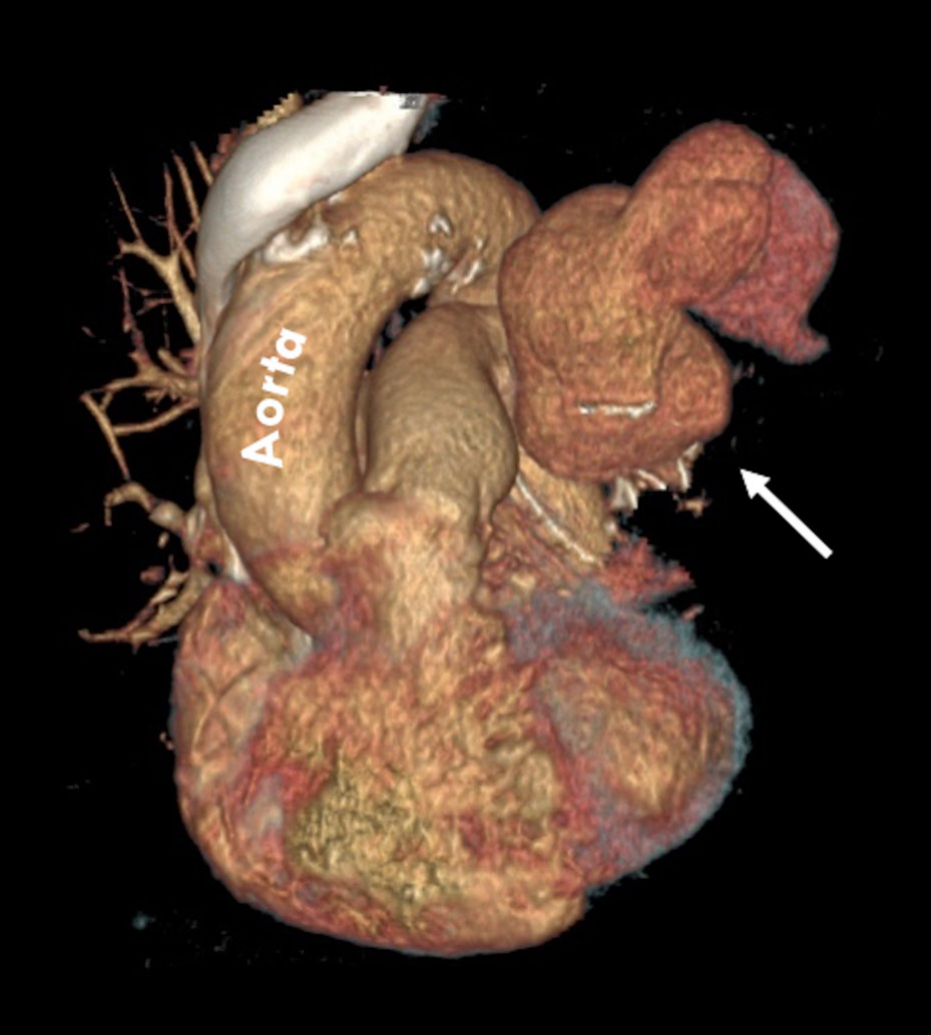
Computed tomography angiography with three‐dimensional reconstruction shows a large contrast‐filled outpouching adjacent to the left pulmonary artery (white arrow).
